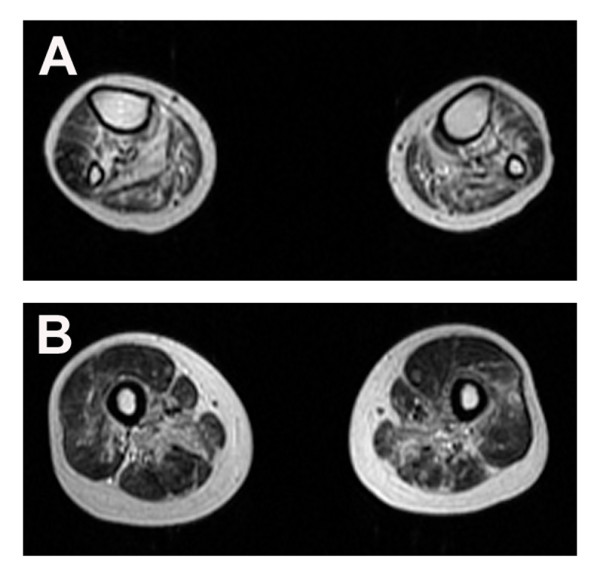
Muscle magnetic resonance imaging. T2-weighted magnetic resonance images of the legs (A) and thigh (B) show severe muscle atrophy and increased signal intensities in the tibialis anterior and posterior, adductor longus and magnus, and biceps femoris muscles.
